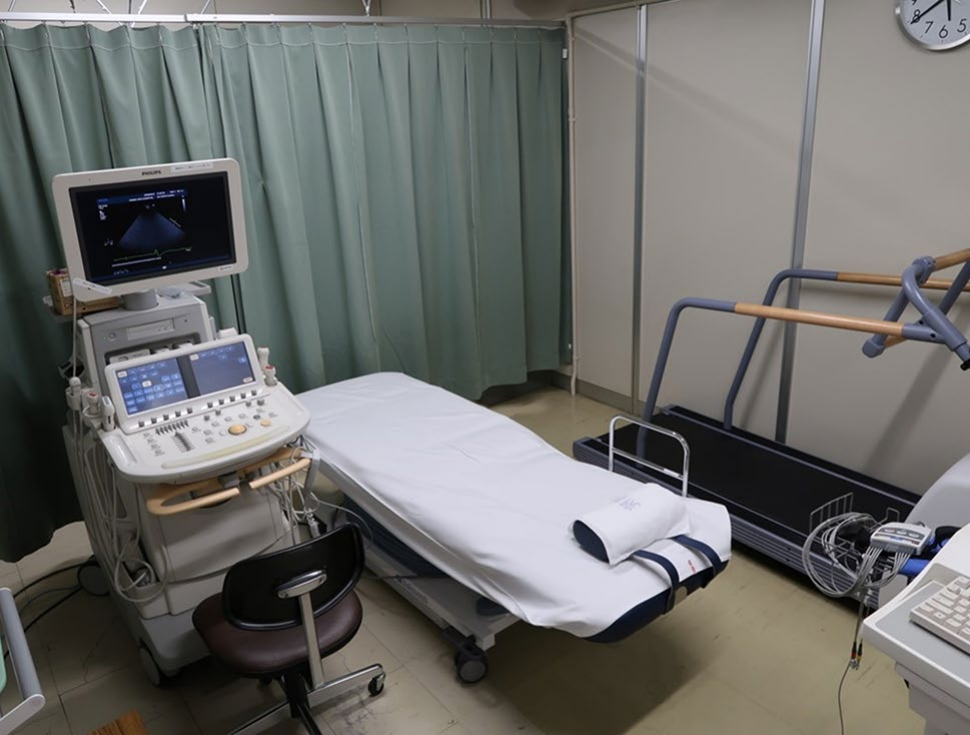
Treadmill exercise testing room (Kindai University Hospital). The treadmill, bed, and echocardiographic system should be arranged as compact as possibleParasternal short-axis view, which contains the perfusion territories of all three coronary arteries, is critical in the exercise stress echocardiographic assessment of the wall motion; yet, oblique sections can be a cause of false-positive or false-negative results. An example: in the case of a long-axis view obtained by directing the beam through a lower intercostal space (Fig. 5a), the short-axis view gained by rotating this long-axis view by 90° results in an oblique section (Fig. 5b). In this section, the anteroseptal region is visualized close to the apex and the posterior wall more basally. Hence on visualizing the posterior wall, because of the influence of the mitral annulus, the inferior wall area may be misinterpreted as a site where there is decreased regional wall motion (arrow, Fig. 5c).
